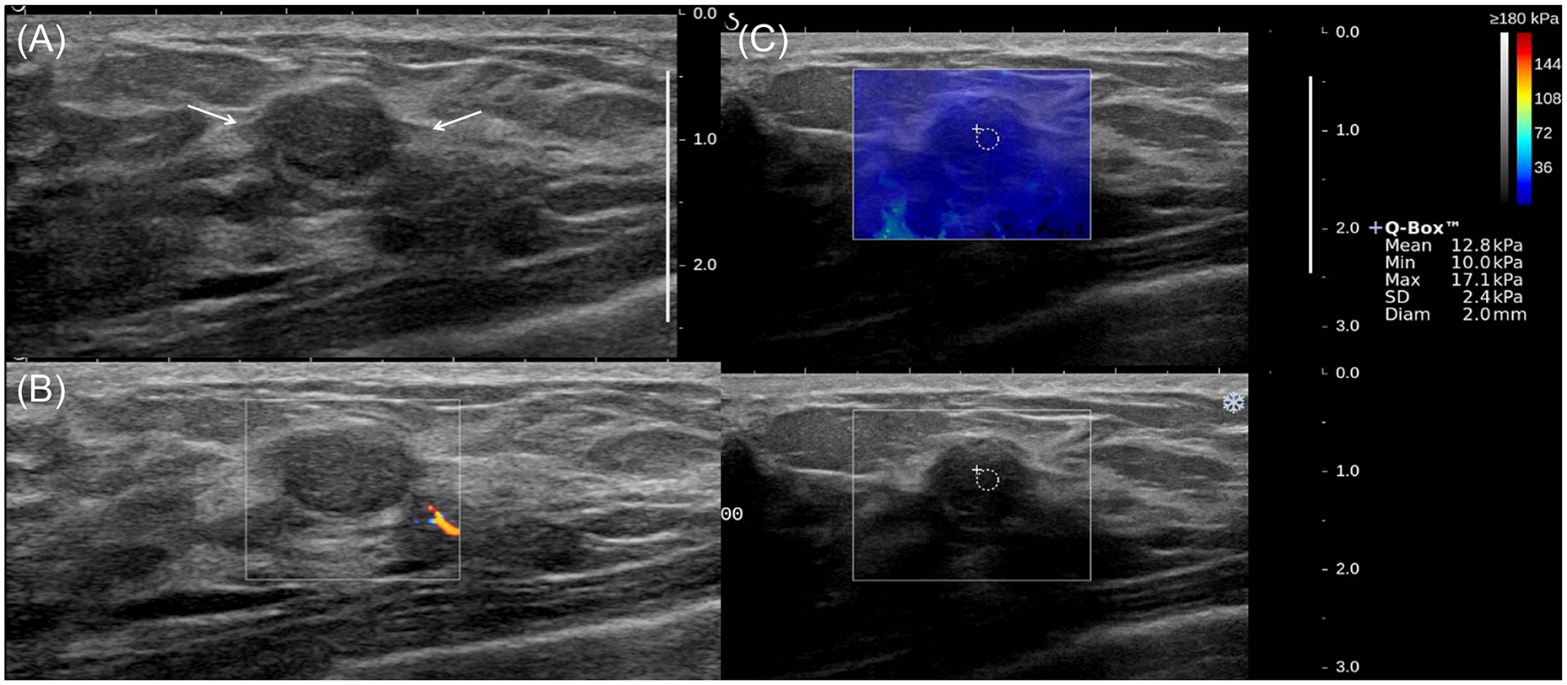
A 36-year-old woman diagnosed with equivocal fibroepithelial lesion by US-guided biopsy, which was confirmed as fibroadenoma on surgical excision. (a) The B-mode US image shows a 1.4-cm breast mass with BI-RADS category 4a (arrows), (b) the color Doppler US image shows low vascularity, and (c) the SWE image shows E_mean_ of 12.8 kPa and E_max_ of 17.1 kPa.
